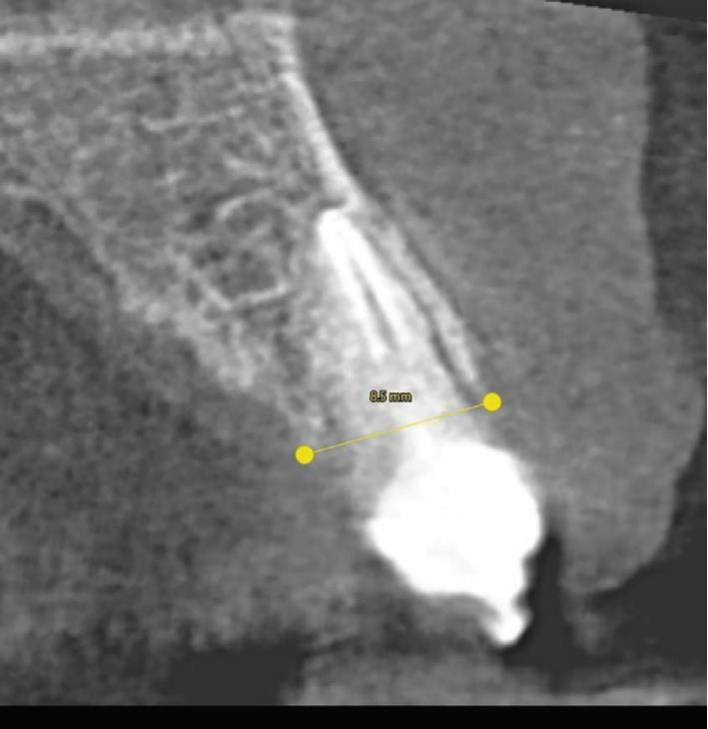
Preoperative (T0) cross-sectional CBCT view showing the measurement line drawn between buccal and palatal cortical plates at the level of the alveolar crest.
